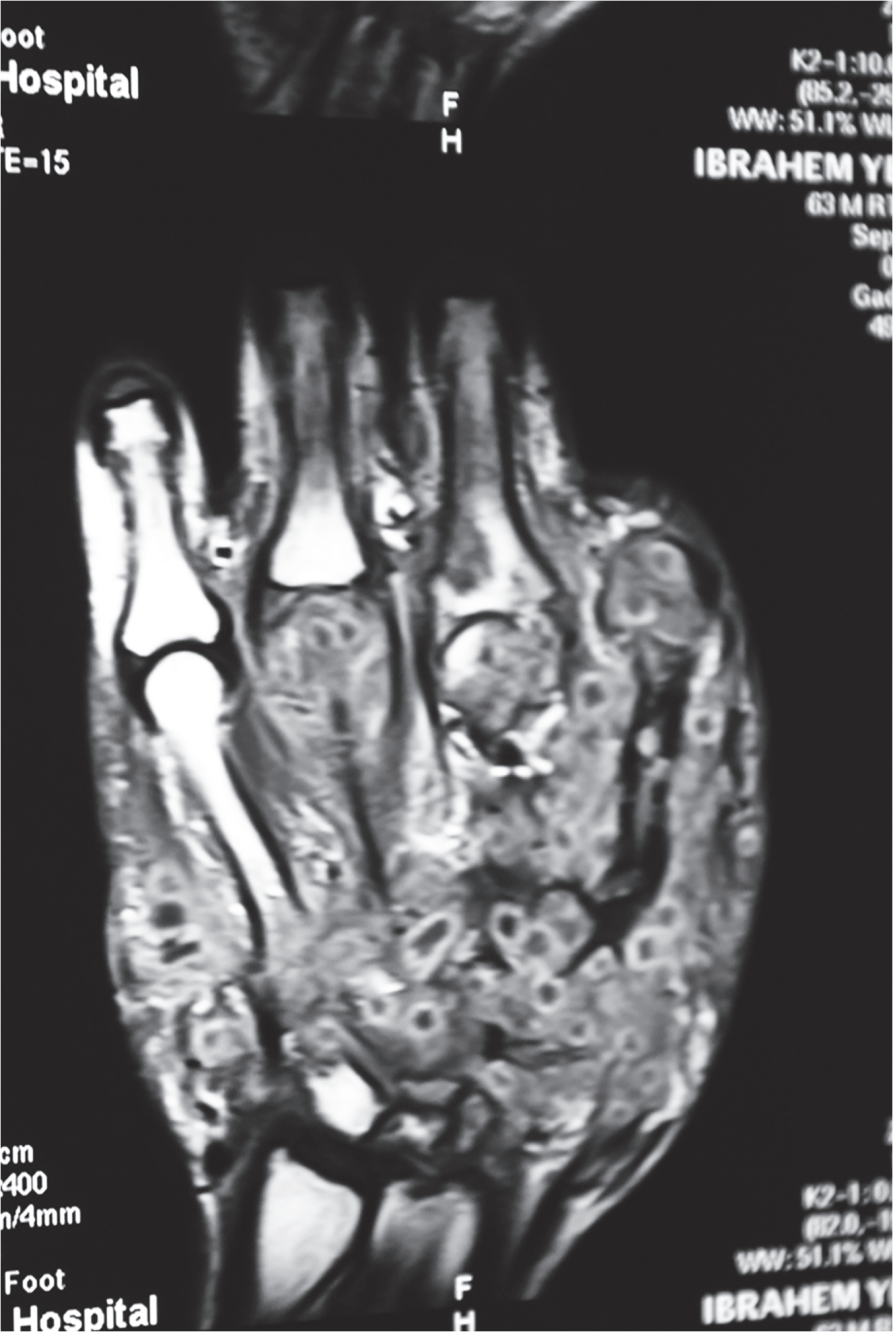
Showing hand MRI with an extensive eumycetoma lesion infiltrating and obliterating the subcutaneous tissue planes. There are multiple low signal intensity fungal grains, bone marrow oedema, and a dot-in-circle sign.
